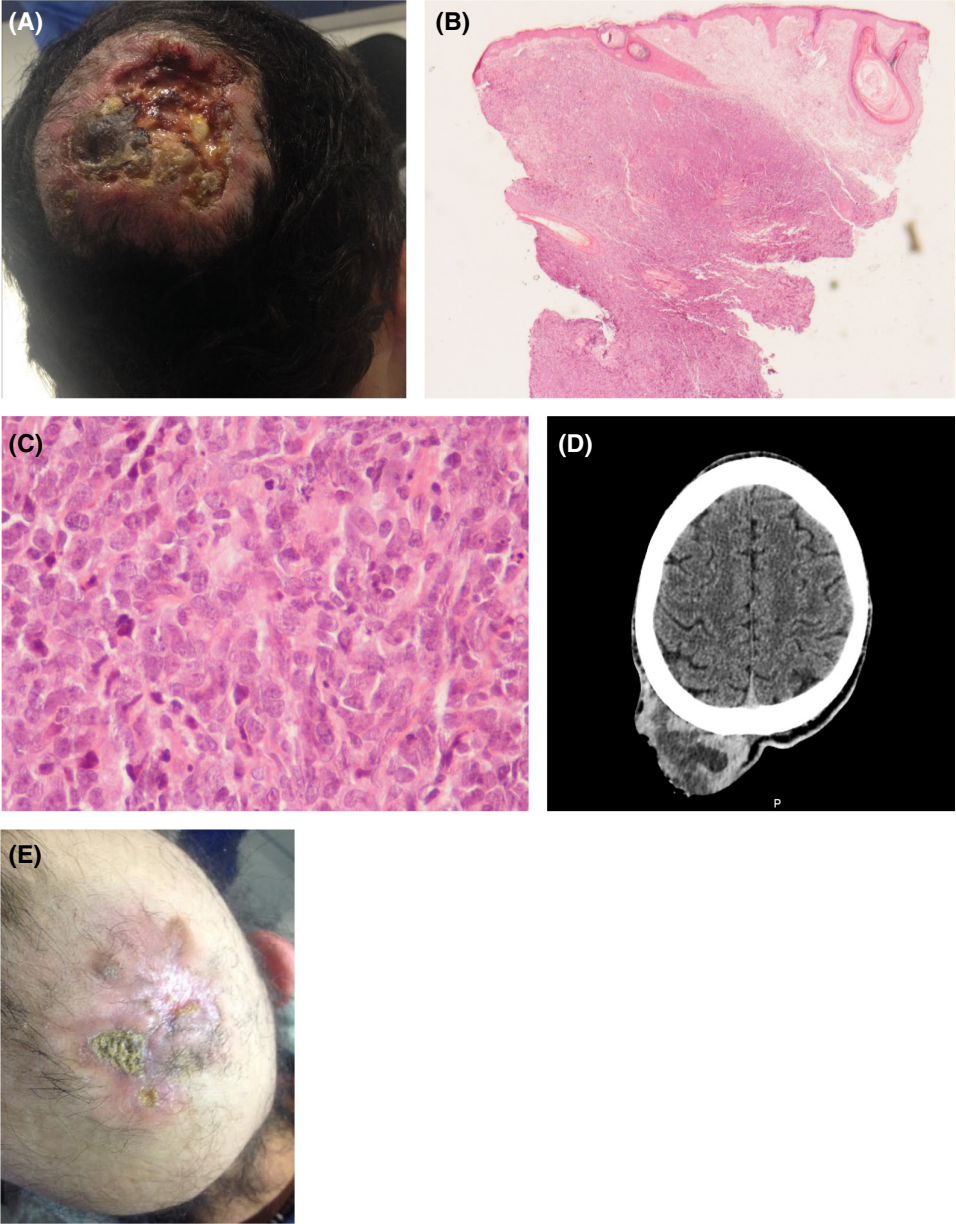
(A) ‐ swelling lesion on the scalp. (B) ‐ diffuse lymphoid dermal and hypodermic infiltration of predominantly large centroblastic B cells (HE, x20). (C) ‐ mixed medium to large cells, predominantly centroblastic (HE, ×400). (D) ‐ brain computed tomography showing a soft tissue tumor on the right parietal region (63x33mm), with no evidence of bone invasion. (E) ‐ scalp lesion after 2 cycles of chemotherapy.
